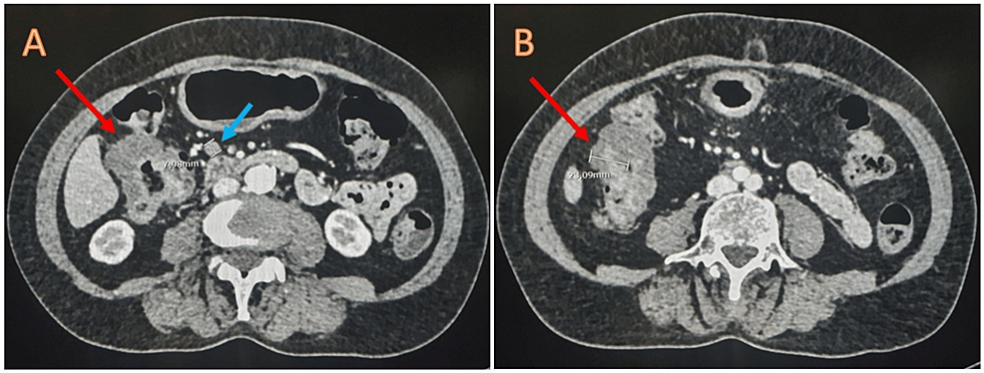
Contrast-enhanced abdominal CT (axial view). (A) The concentric thickening of hepatic flexure (red arrow), occluding 70% of the lumen, can be seen. The light blue arrow shows an 8-mm paracolic lymph node. (B) The thickest zone measured 23 mm (red arrow) at the ascending colon, obstructing 90% of the bowel lumen.
